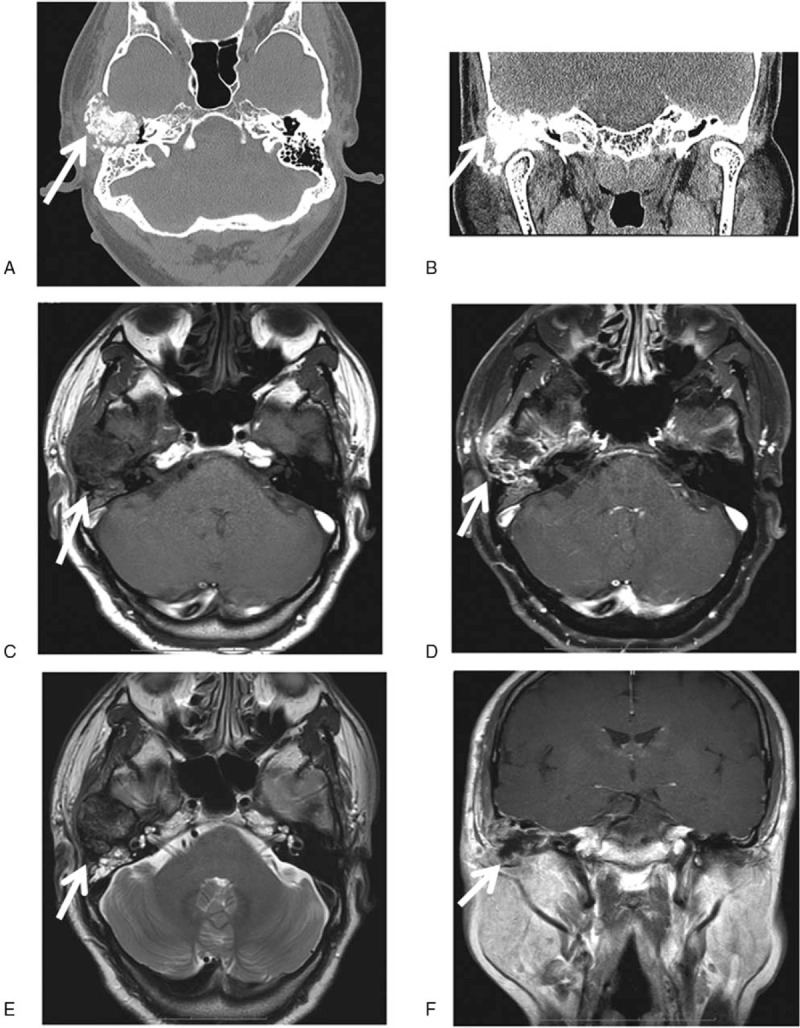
Temporal bone CT scan and MRI results of the CMF patient: (A) CT axial plane images, (B) CT coronal scan. The results display the right temporal bone plate and tympanic plate presenting with swelling, cloudy flocculent ground-glass opacity, clear boundary, thin bone in the middle of the cranial fossa, low continuity, and involving the temporom and ibular joint. The lesion exhibited hypointensity and presented as an oval soft tissue shadow on the axial T1-weighted image (C, arrow) and T1-weighted image of the coronal aspect (F, arrow). Heterogeneous hyperintensity on the axial T2-weighted image (E, arrow) and T1-enhanced weighted image of the axial position shows ring enhancement (D, arrow). CMF = chondromyxoid fibroma, CT = computed tomography, MRI = magnetic resonance imaging.
